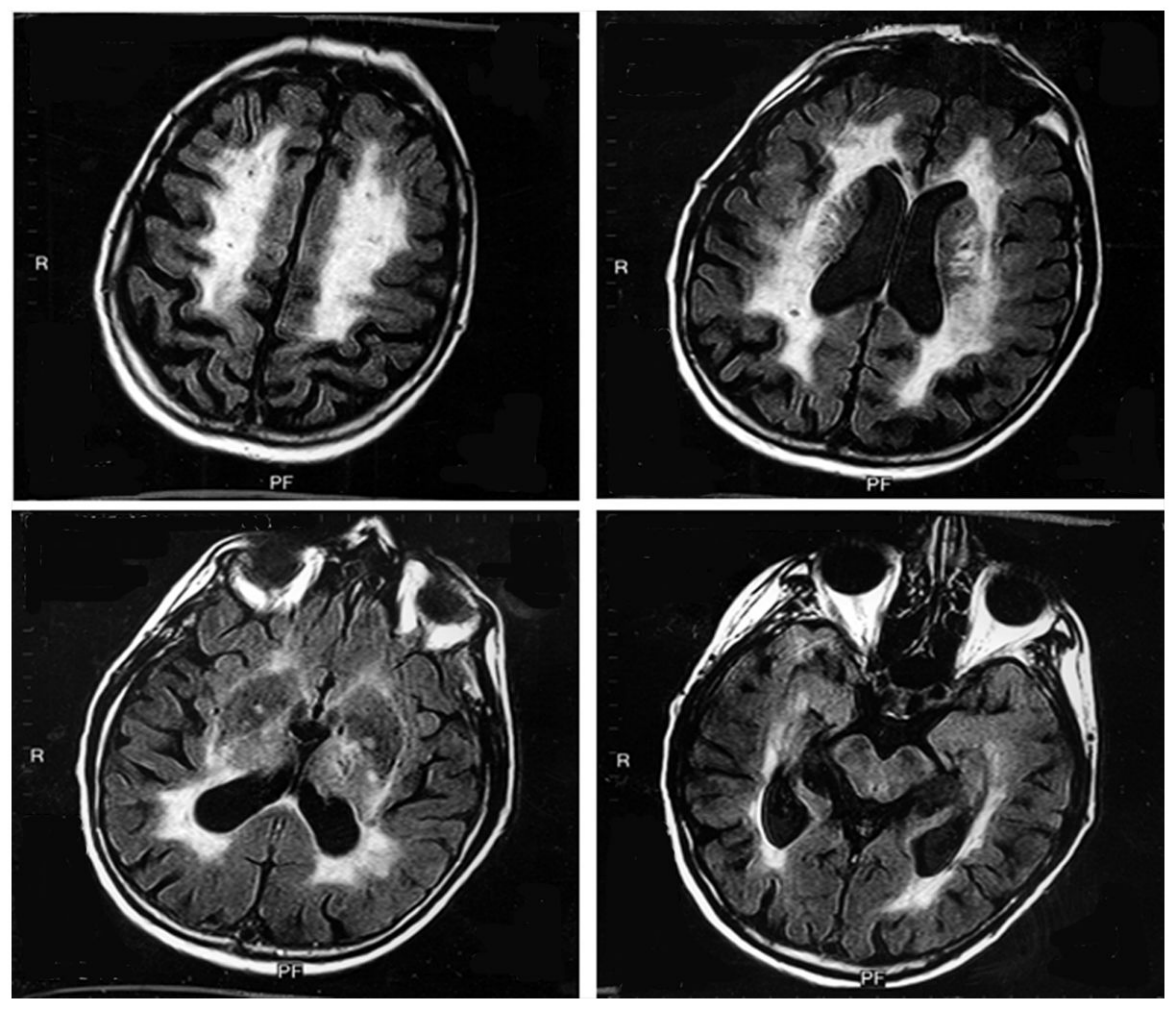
Bilateral small chronic infarcts and patchy and confluent areas of leukoaraiosis seen on fluid attenuated inversion recovery (FLAIR) MRI on patient with NOTCH3 p.R558C mutation.
